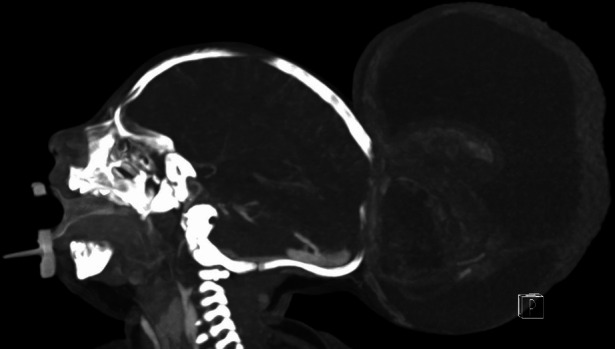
Preoperative CT-angiography reveals the absence of a vein of Galen complex and a hypoplastic transverse and sigmoid sinus on the left side
